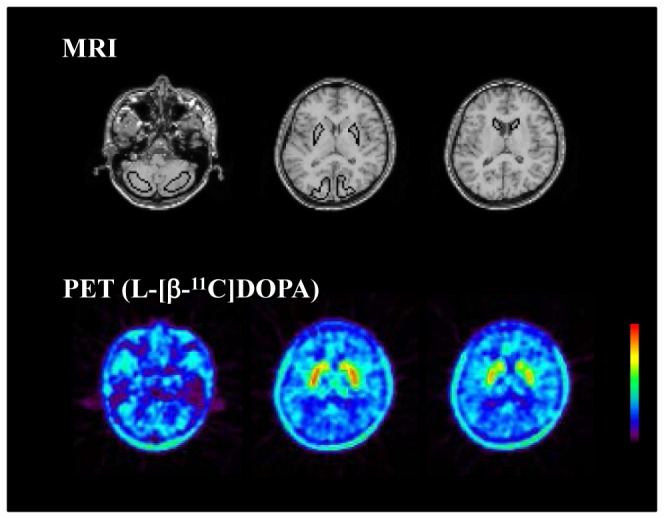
Regions of interest (ROIs) drawn on coregistered MR images. ROIs are defined for the cerebellar cortex, putamen, caudate head, and occipital cortex. Typical PET summation images of frames between 29–89 min after intravenous injection of L-[β-^11^C]DOPA for baseline study are also shown.
